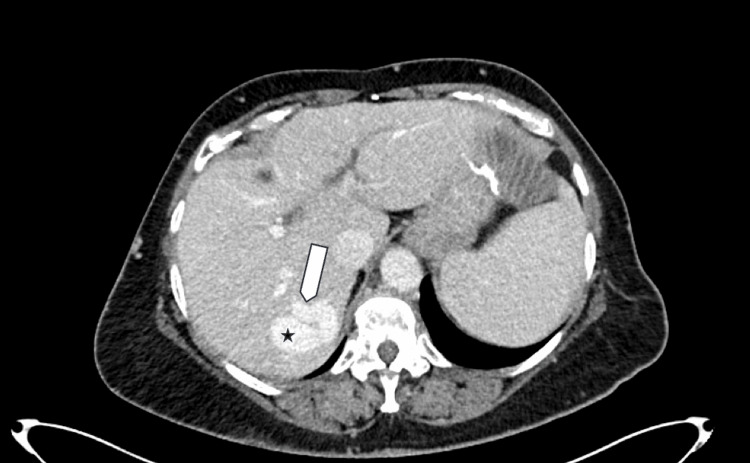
Portal venous-phase axial CT showing a portosystemic shunt The arrow indicates the feeding right portal vein, and the star marks the draining right hepatic vein.
